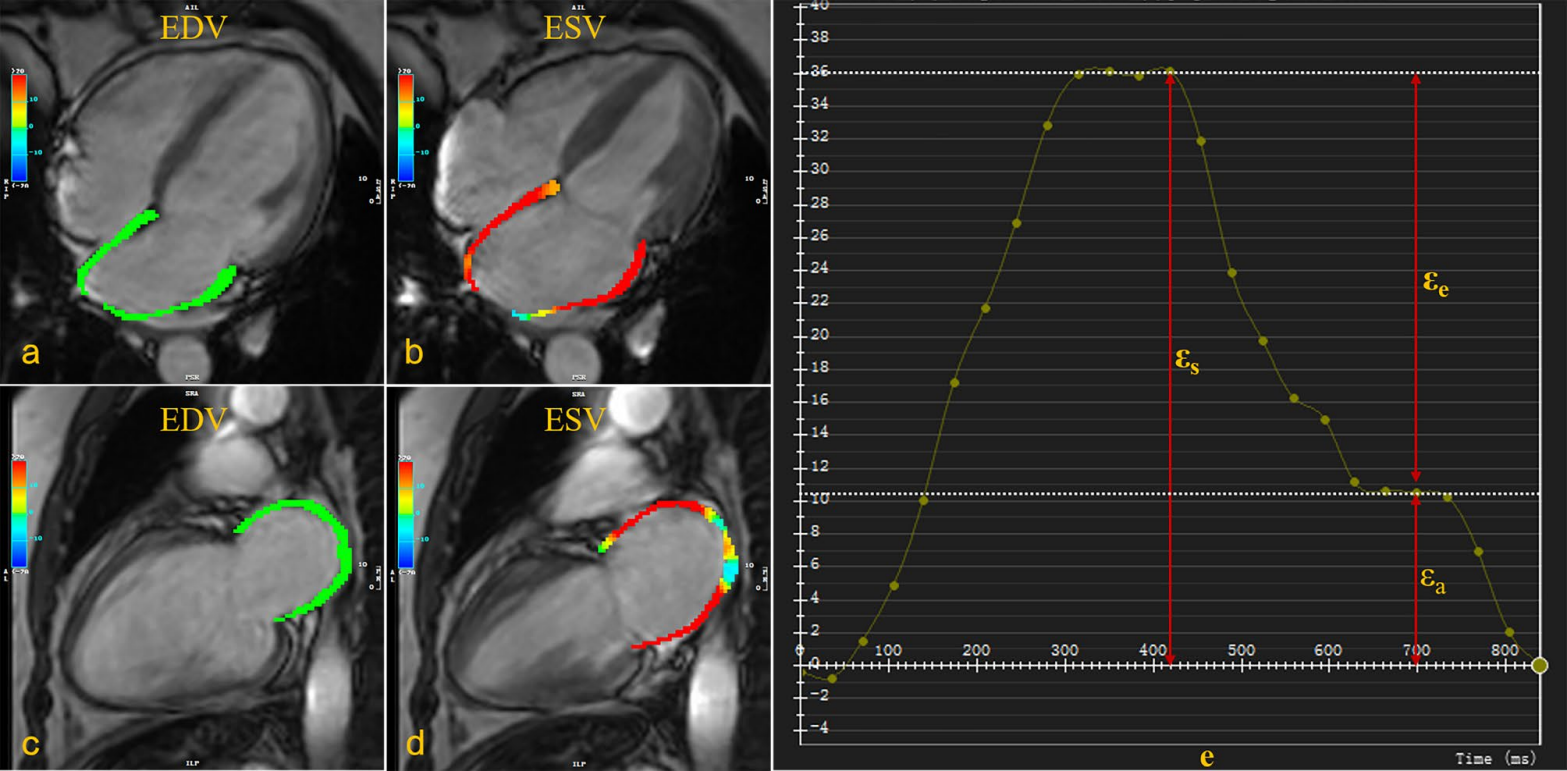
Representative Images and Plot of MRI-Derived Left Atrial Longitudinal Strain. (a) Four-chamber view of left atrial longitudinal strain at end-diastolic phase; (b) Four-chamber view of left atrial longitudinal strain at end-systolic phase; (c) Two-chamber view of left atrial longitudinal strain at end-diastolic phase. (d) Two-chamber view of left atrial longitudinal strain at end-systolic phase; (e) Plot of left atrial phasic longitudinal strain, including reservoir strain (ε_s_), conduit strain (ε_e_), and booster strain (ε_a_)
